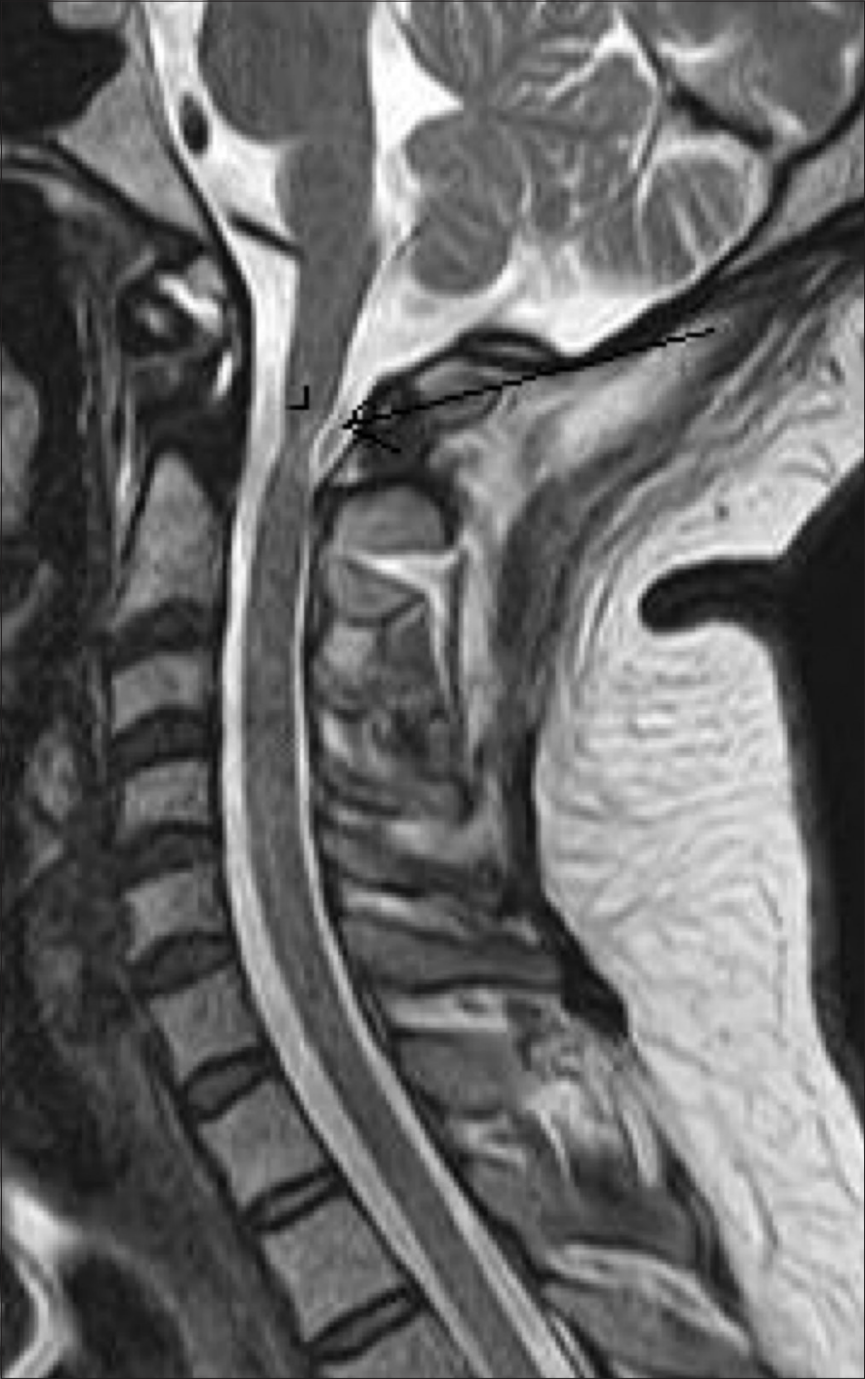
Sagittal T2W MRI image shows reduced caliber of the cervical spinal cord (arrow) with a hyperintense intramedullary signal (arrowhead) at the C1-C2 level
